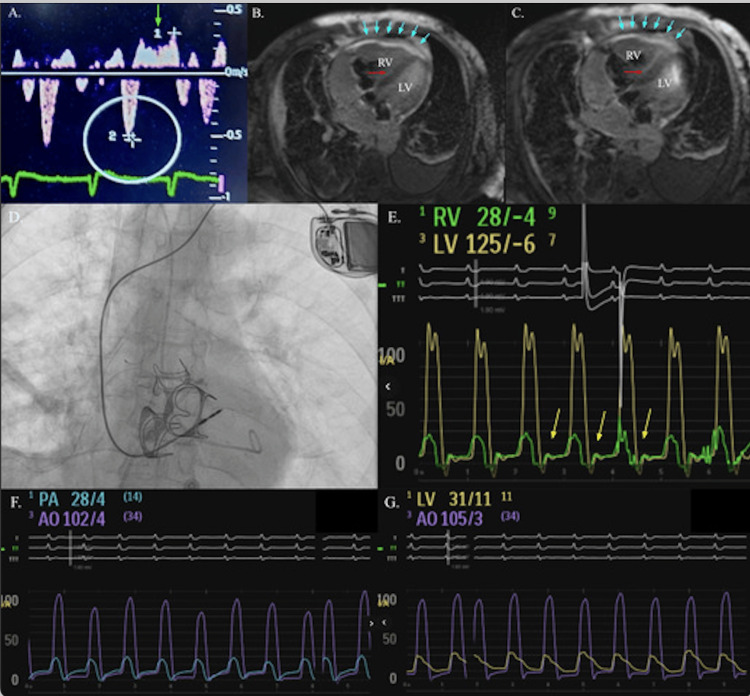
Multimodality assessment demonstrating constrictive physiology. Findings are grouped by their diagnostic weight for constrictive pericarditis. Supportive (non-invasive) findings. A: Doppler echocardiography showing prominent expiratory hepatic vein flow reversal (green arrow). B-C: Cardiac magnetic resonance imaging revealing pericardial thickening (blue arrows) with septal bounce (red arrow). Diagnostic (invasive) findings. D: Fluoroscopic view of catheter positioning during the haemodynamic study. E: Simultaneous right- and left-ventricular pressure tracings showing the dip-and-plateau ("square-root") sign in early diastole (yellow arrows). F-G: Simultaneous pressure tracings demonstrating marked respiratory discordance between left ventricular and aortic pressures, consistent with enhanced ventricular interdependence. The invasive tracings (E-G) were considered diagnostic of constriction, whereas the echocardiographic and CMR findings (A-C) were supportive.
